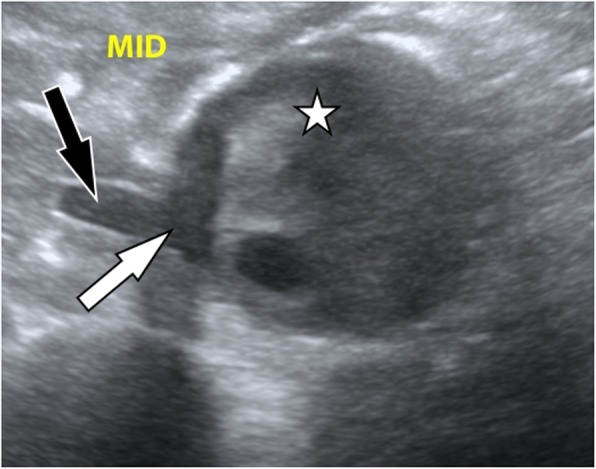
Ultrasonographic transverse view of the abdominal aortic aneurysm with an inhomogeneous appearance of the luminal thrombus (star), a focal disruption of the luminal thrombus and the outer wall of the aneurysm (white arrow), and a para-aortic hypoechoic area (black arrow)
